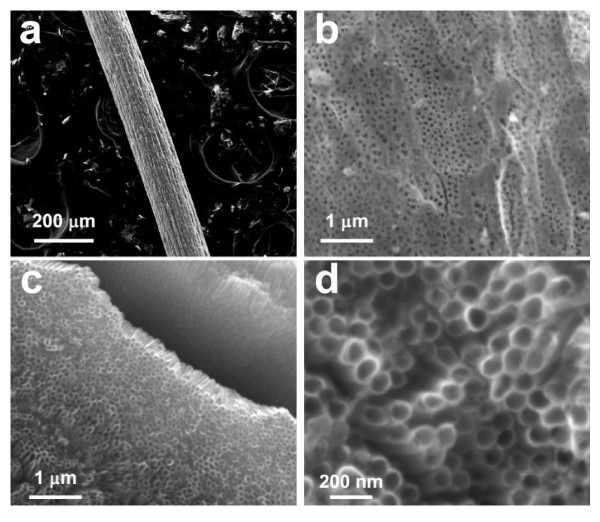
Characterization of surface topography (a) FESEM image of an anodized Ti wire with a diameter of 127 μm; (b) FESEM image of outer surface of an as-anodized Ti wire. (c) Large-scale FESEM image of an anodized Ti wire after chemical etching or ultrasonication. All exposed TiO_2 _nanotubes were open-ended and the broken part in the porous ATO membrane shows clearly the side-view of the nanotubes. (d) Enlarged FESEM image of individual TiO_2 _nanotubes which average diameter is about 105 nm.
